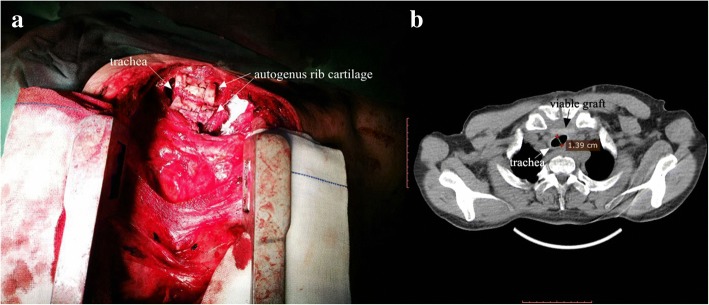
Tracheal suspension with autogenous rib cartilage (a intraoperative photograph of finished scaffold, b postoperative CT image)
